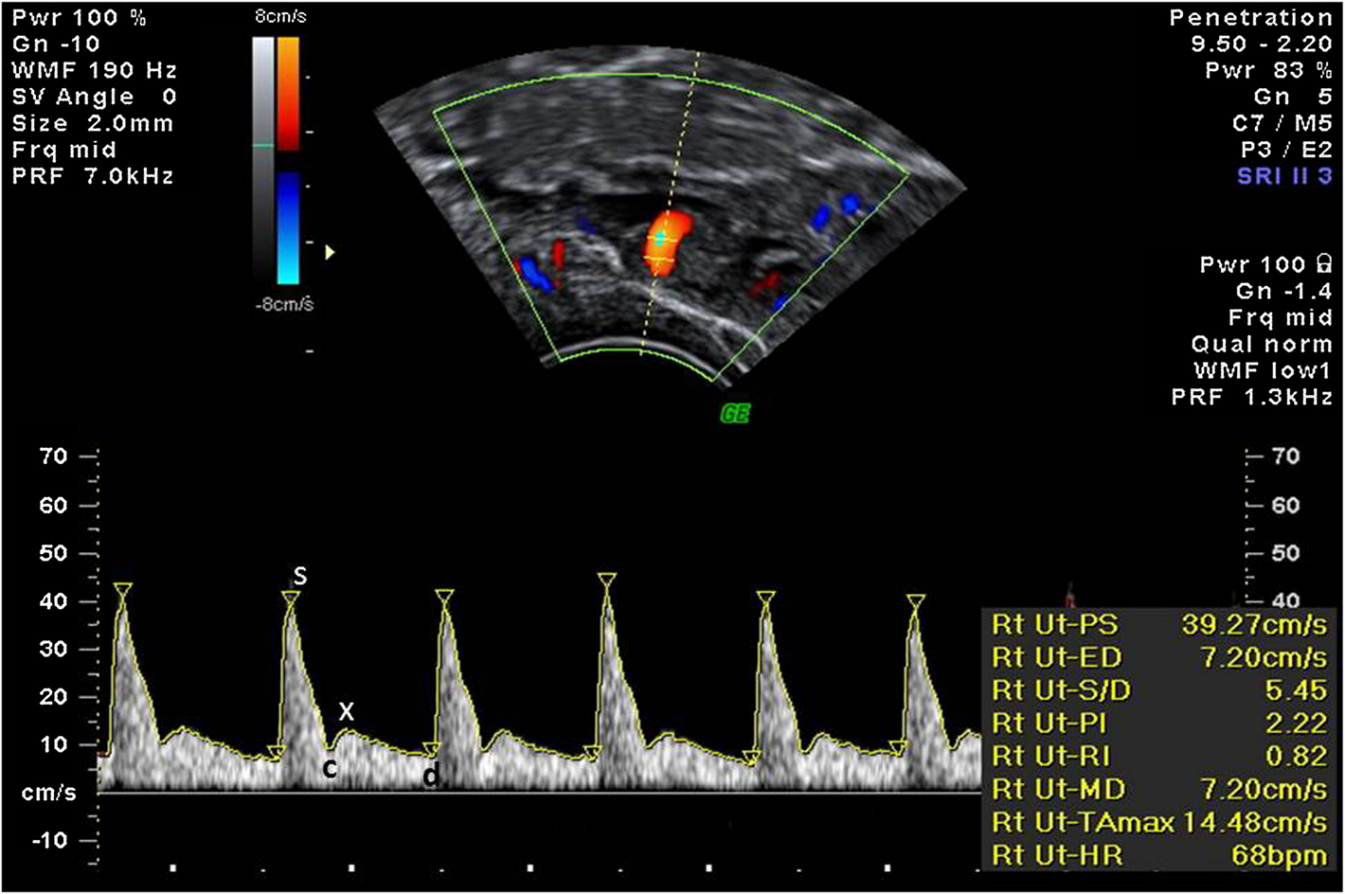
Doppler spectra of uterine artery flow. Pulsatility index (PI) is used as a measure of impedance of the flow of blood distal to the sampling point and is automatically calculated according to the formula $$ PI=\frac{\left(s-d\right)}{mean} $$ where *s* is the peak *d* is the minimum and the average is the *mean* maximum Doppler shift frequency over the cardiac cycle. Resistance index (RI) is automatically calculated using the formula $$ RI=\frac{\left(s-d\right)}{s} $$
